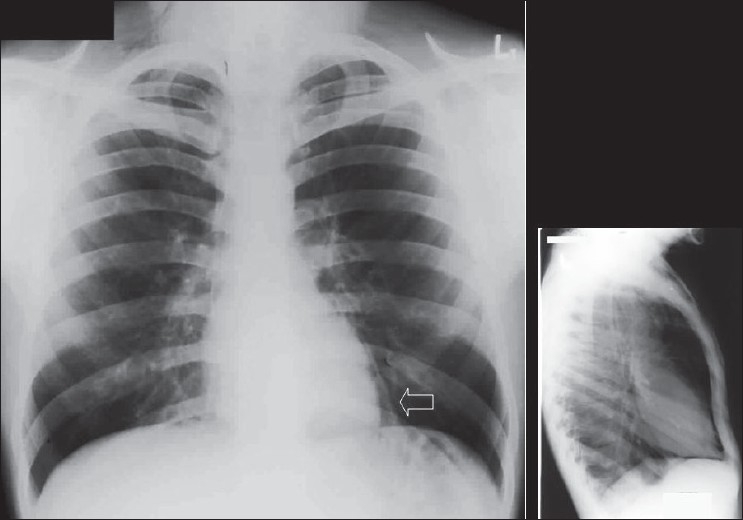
Frontal and lateral radiographs depicting a pneumopericardium
